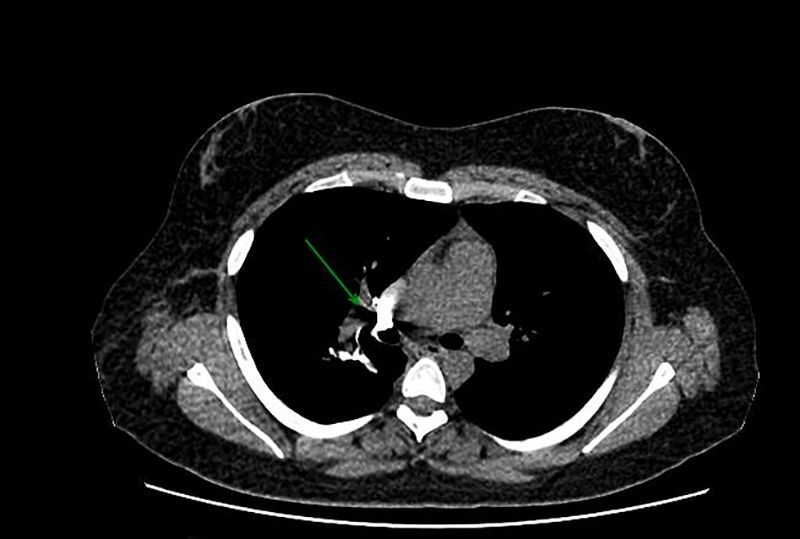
Non-contrast chest CT scan showing signs of surgical manipulation in the right pulmonary hilum, with clips and peri-hilar metallic suture, with slight prominence of the hilar region.
